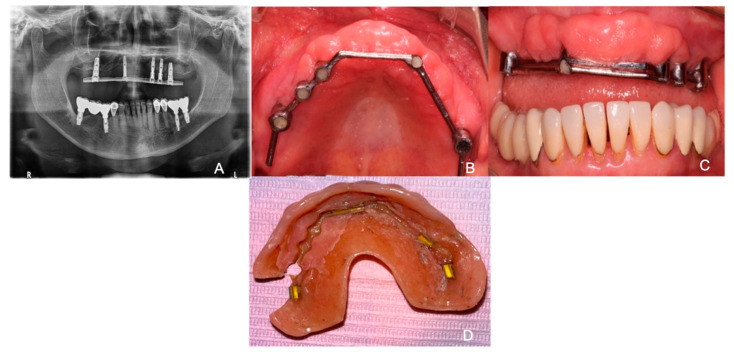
(A) Panoramic radiographic image displaying the failed bar-retained attachment. (B) Bottom of the palate with white appearance and generalized inflammation. (C) Anterior part of maxilla with multinodular oval-shaped tissue. (D) Interior part of the overdenture with presence of plaque and a fracture in the posterior left side.
